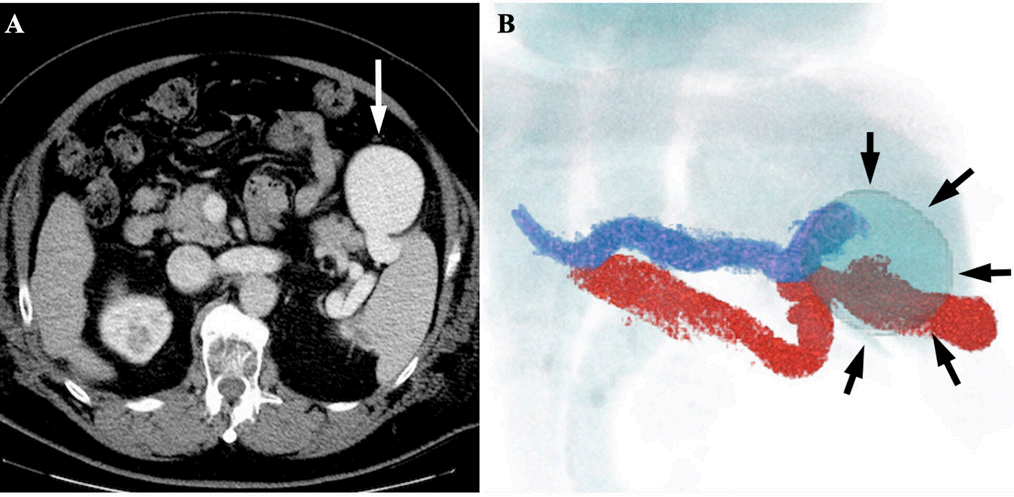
Contrast-enhanced CT image of the portal phase. (A) Axial; (B) 3DCT. (A) Axial image shows a dilated and tortuous splenorenal shunt with a large venous aneurysm (65 mm × 55 mm × 58 mm) (*white arrow*) in the hilus of the spleen. (B) On 3DCT, venous aneurysm of the splenorenal shunt (*black arrows*) and feeding and draining veins are clearly recognizable. The feeding vein is the splenic vein (blue line) and the draining vein is the left renal vein (red line).
